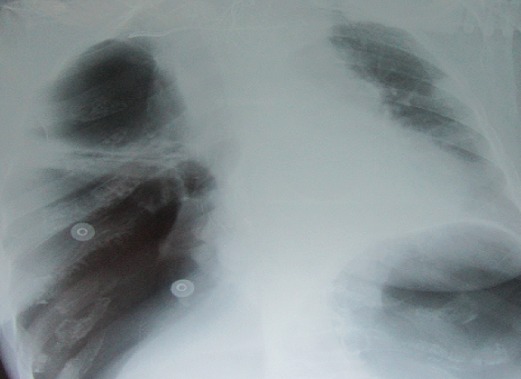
Radiographie du thorax montant un pneumothorax droit de grande abondance refoulant le poumon au niveau médiastinal
